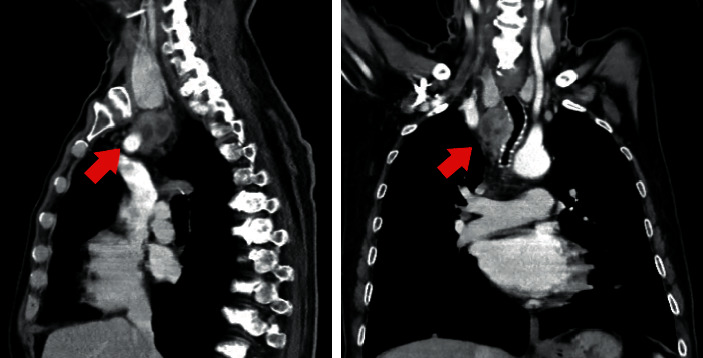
Neck and chest CT. The sagittal image revealed a mediastinal mass (41 × 29 mm) with clear demarcation form the right lobe of the thyroid gland. The coronal image shows the mediastinal mass interposing between the trachea and supra-aortic vessels.
